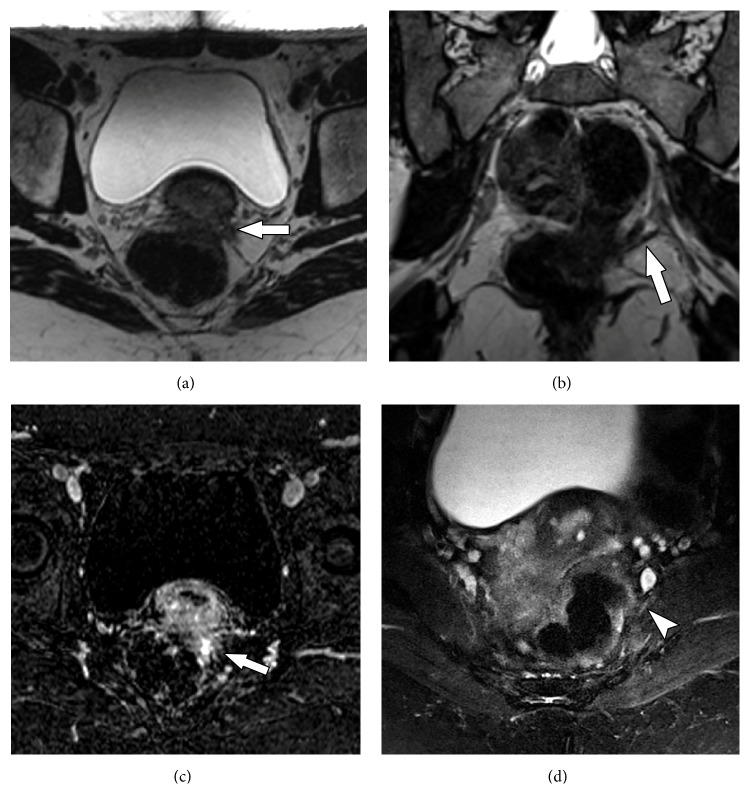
31-year-old female subject, presenting numbness and tingling on the left lower extremity for three months. Past medical history of endometriosis. MRN images on axial T2 isotropic (a), coronal T2 isotropic (b), axial T1 VIBE subtraction (c) show deep endometriosis in the uterine retrocervical region, extending to the left paracervical space maintaining an intimate relationship with the pelvic root path (solid arrows) and axial T2 FLAIR (d) demonstrates slight change of the emerging left S2 root signal intensity with mild contrast enhancement (arrowhead).
